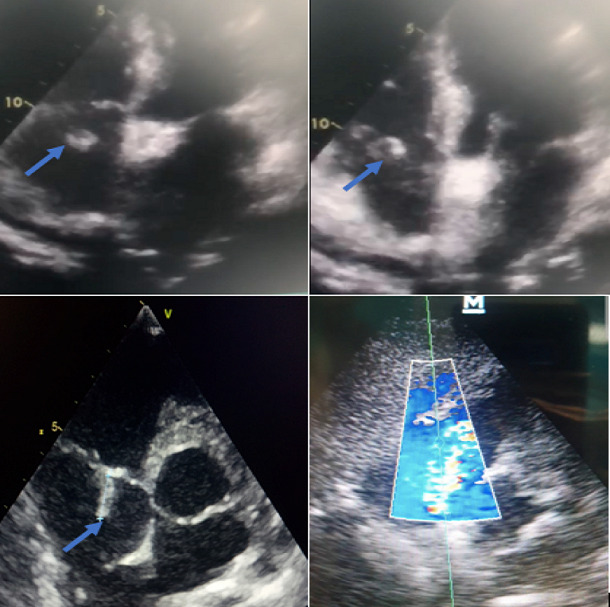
Transthoracic echocardiography images showing oscillating vegetation attached to the anterior leaflet chordate of the tricuspid valve (blue arrow) with a moderate tricuspid regurgitation
